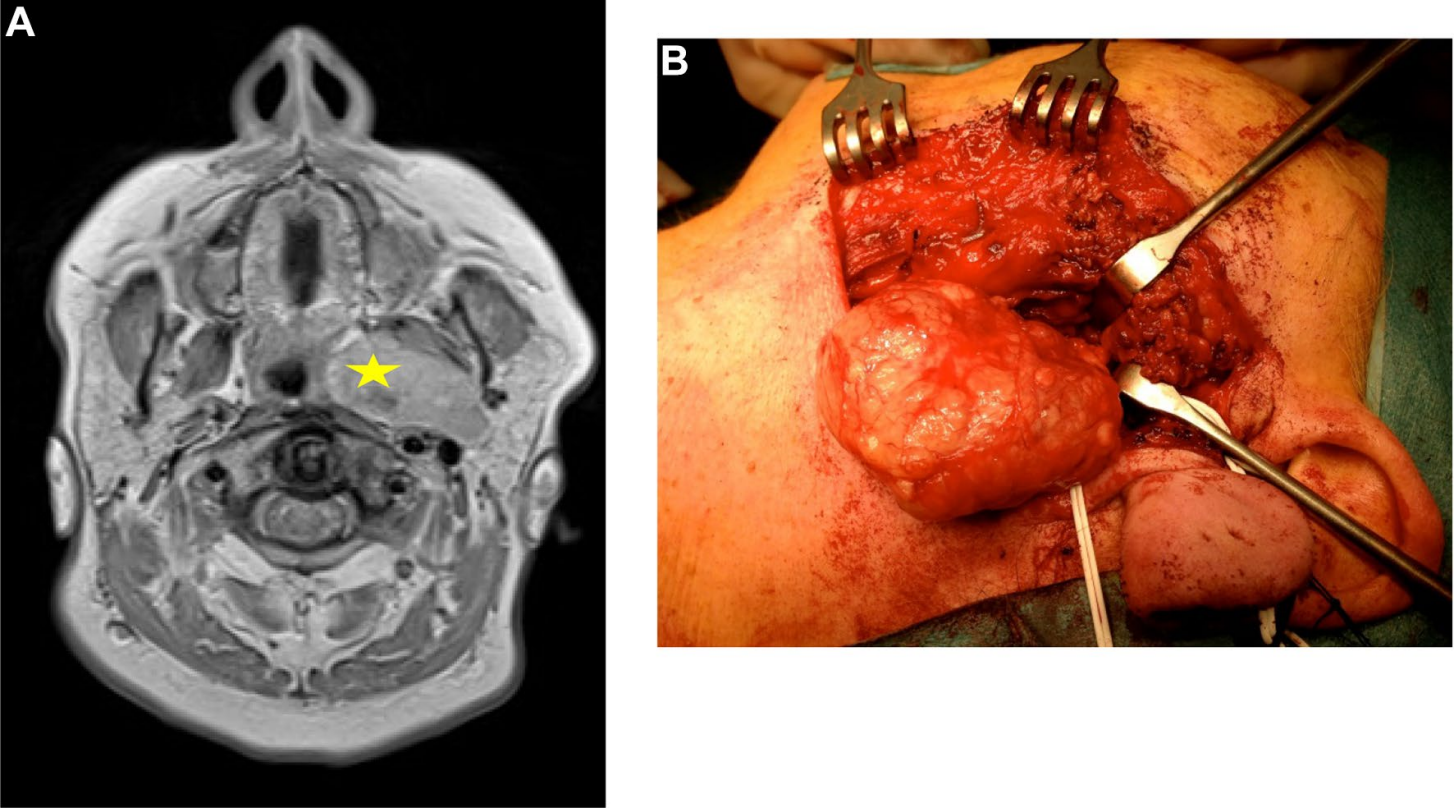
a Axial T2-weighted image of a pleomorphic adenoma (star) extending from the deep lobe of the left parotid. b Intra-operative image of removal through a cervical—transparotid approach. The stylomandibular ligament was cut to allow delivery of the tumor through the neck. The superficial lobe was repositioned after mobilization for access
